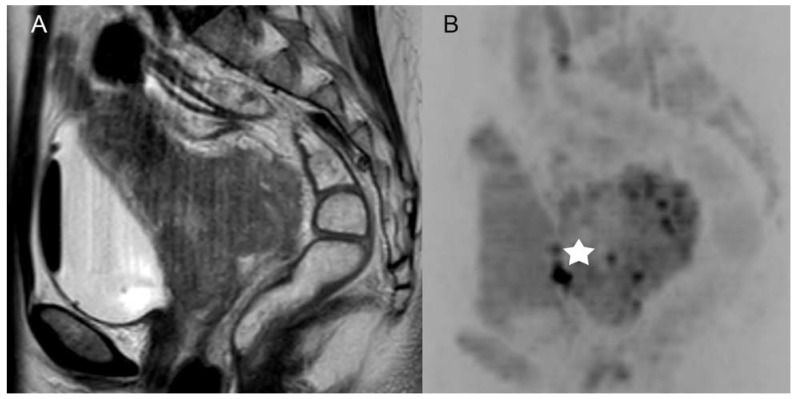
Example of a patient with a FIGO IV cervical carcinoma with a biopsy proven infiltration of the mucosa of the bladder which had not been recognized by both readers in the standard MRI ((A), sagittal T2-weighted scans without fat saturation), but was recognized in DWIBS (B), marked with a white star (*).
